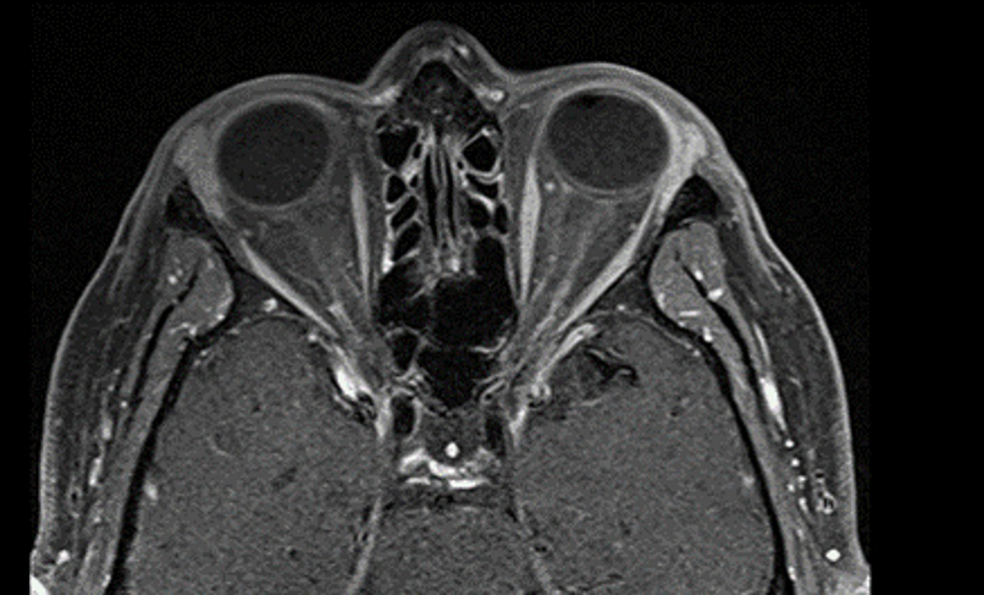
MRI orbits MRI orbits reveal mild inflammatory changes of the left conjunctiva, anterior chamber, anterolateral sclera, left lacrimal gland, and paranasal sinuses.
